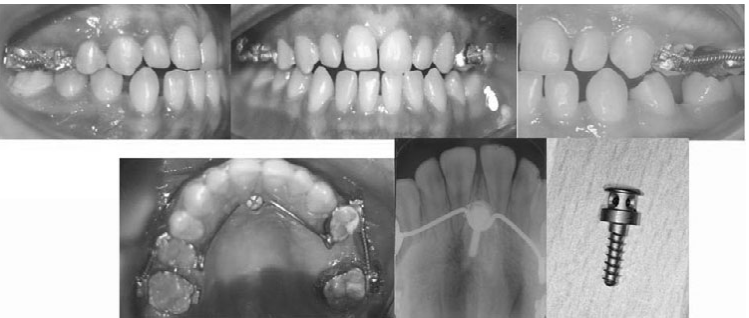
After distalization intraoral photographs and occlusal radiograph of the patient and intraosseous screw.
